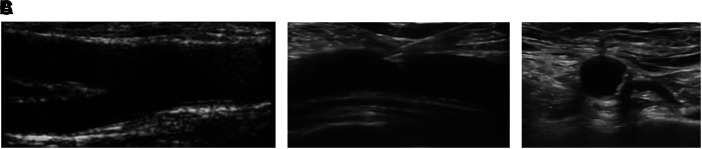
Ultrasound-guided femoral puncture. (*A*) Obtain a longitudinal view of the femoral bifurcation; (*B*) Puncture of the CFA on top of the femoral head with a clear tenting; (*C*) Keep tenting and rotate anti-clockwise the probe and enter the vessel on the anterior wall.
